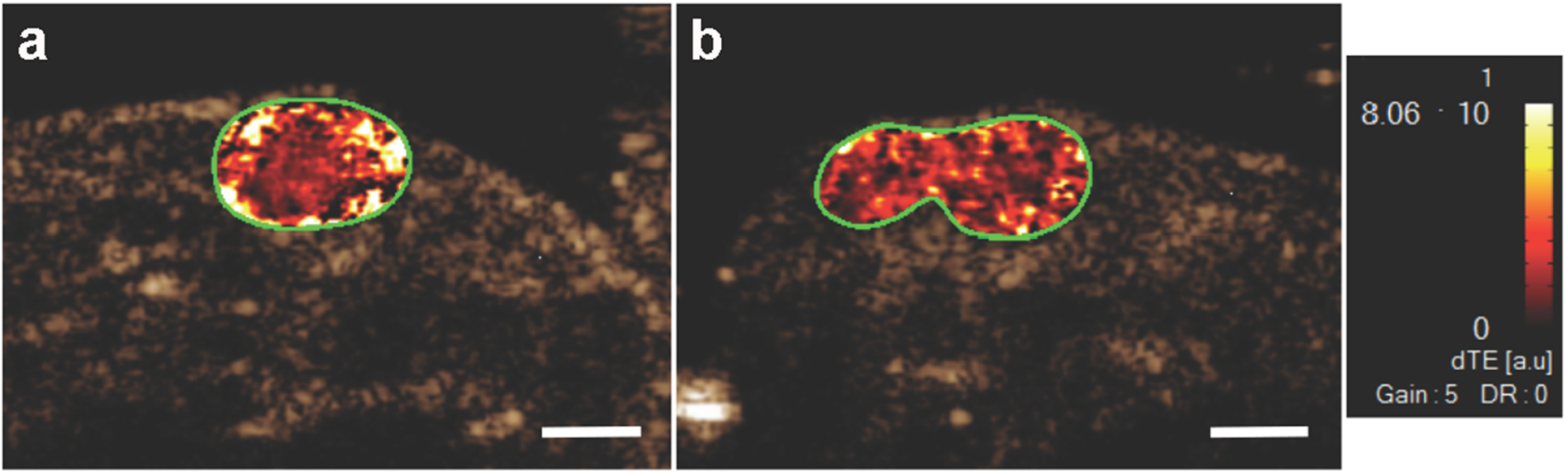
Representative US images showing targeted tumor localization of MB_Tz-TCO_-ARP in PC-3 tumor xenografts. The PSMA^+^ or PSMA^−^tumor were imaged 4 min after intravenous administration of MB_Tz-TCO_-ARP followed by imaging the other tumor. The images show qualitatively higher US signal in the PSMA^+^ tumor (a) compared to the signal found in the PSMA^−^tumor (b). Images are transverse color-coded parametric images overlaid on a non-linear contrast mode US image with whole PC-3 xenograft tumor (green outline) in the field of view. Scale bar = 2mm; dTE = differential targeted enhancement.
